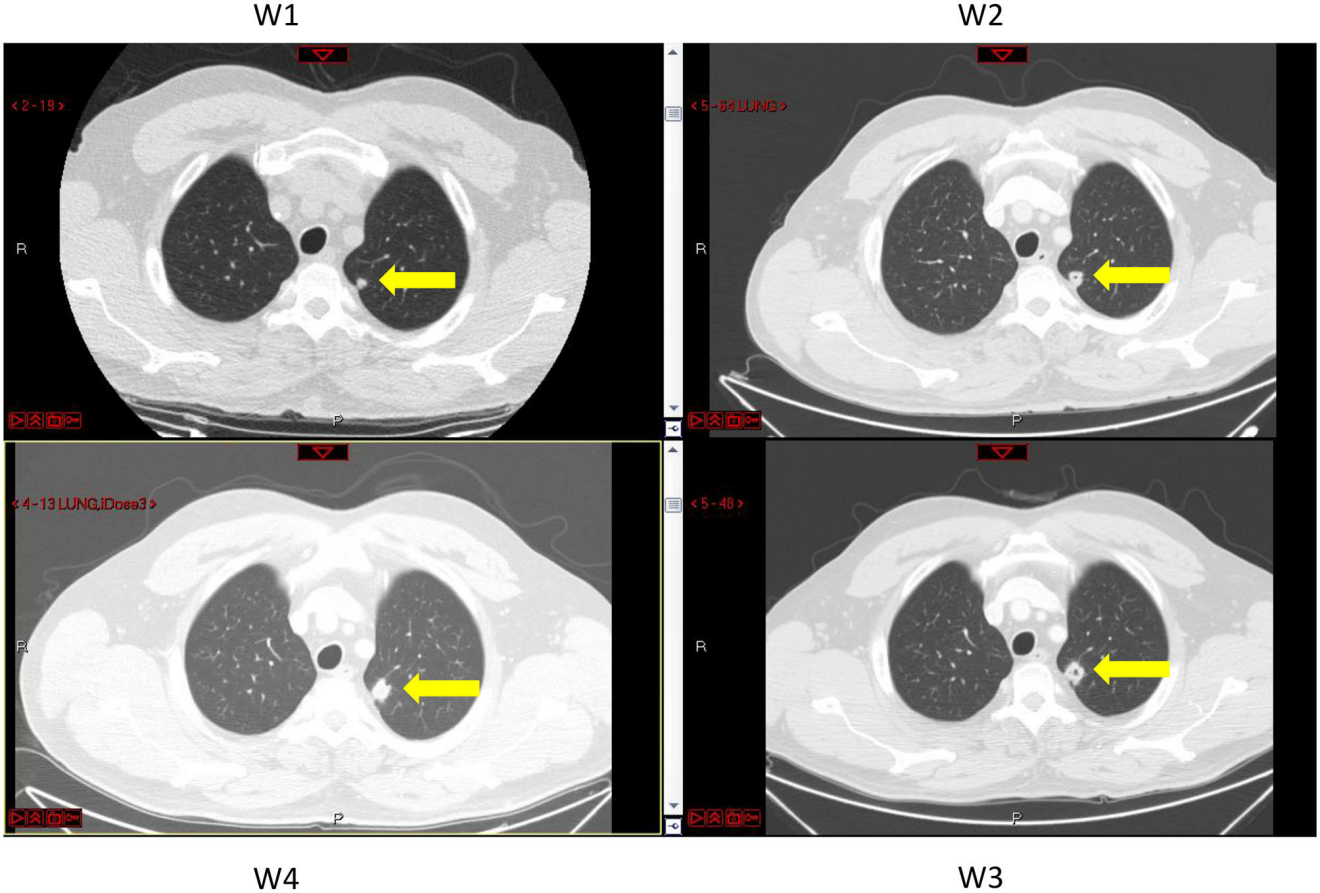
CT images of the lungs taken at different times, marked W1 to W4, at which metastasis volumes were measured (see Figure 1 and Supplementary Table 1). Metastasis #4 is marked by yellow arrows. A pore in the center of the lesion can be seen at times W2 and W3.
